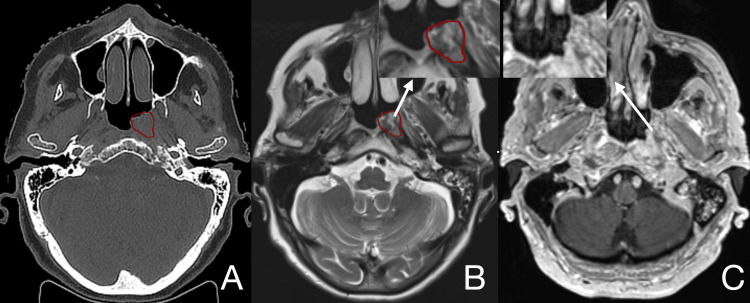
Tumor delineated before radiosurgery in CT (A) and T2 MRI (B), with almost complete regression 18 months after radiosurgery on T1 MRI (C) for the first patient.
